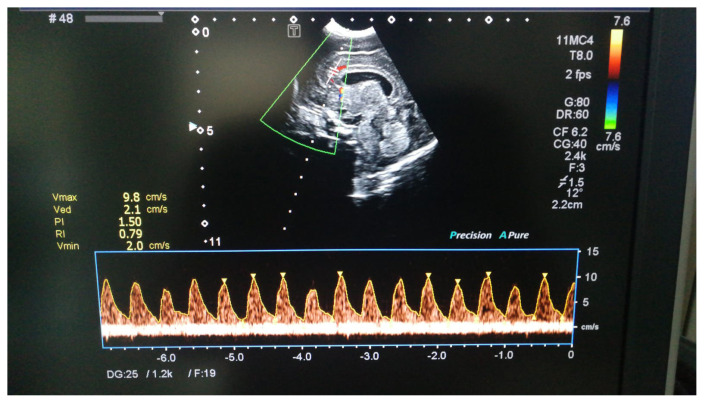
Resistive index (RI) is calculated from Doppler waveforms obtained from anterior cerebral artery with caliper placement in sagittal plane.
